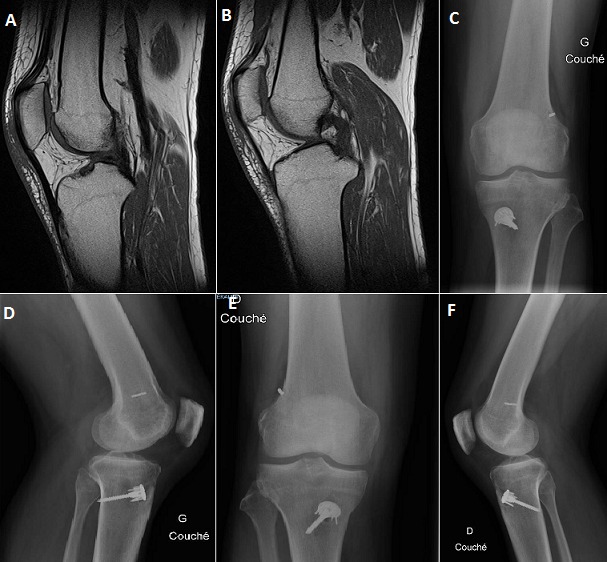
(A) rupture du LCA sur l'IRM du genou gauche; (B): rupture du LCA sur l'IRM du genou droite; (C) bon positionnement du transplant du LCA sur la radiographie de profil du genou gauche; (D) bon positionnement du transplant du LCA sur la radiographie de face du genou droit
